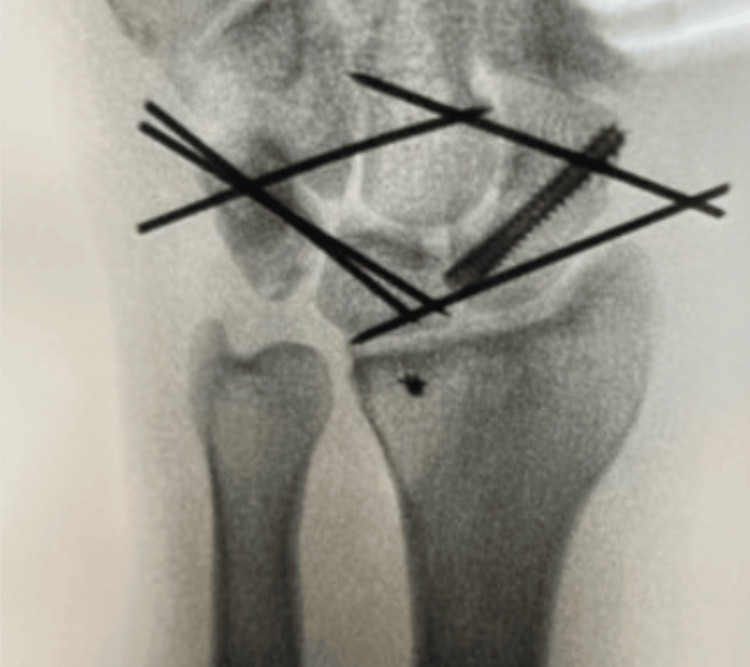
Posteroanterior view of radiograph showing postoperative surgical fixation with a scaphoid screw, pins, and mini anchor.
